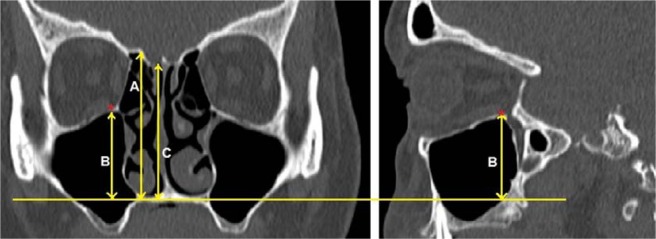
The heights of the ethmoid roof (A), orbital floor (B), and cribriform plate (C) relative to the nasal floor in coronal CT PNS. Each vertical height was measured 90° perpendicular to the nasal floor. To assess the risk of skull base injury using TMS classification, the distances from orbital floor to cribriform plate (OF-CP) and from orbital floor to ethmoid roof (OF-ER) were used. Red asterisk denotes the maximum height of the maxillary sinus which is the level taken as the orbital floor landmark.
